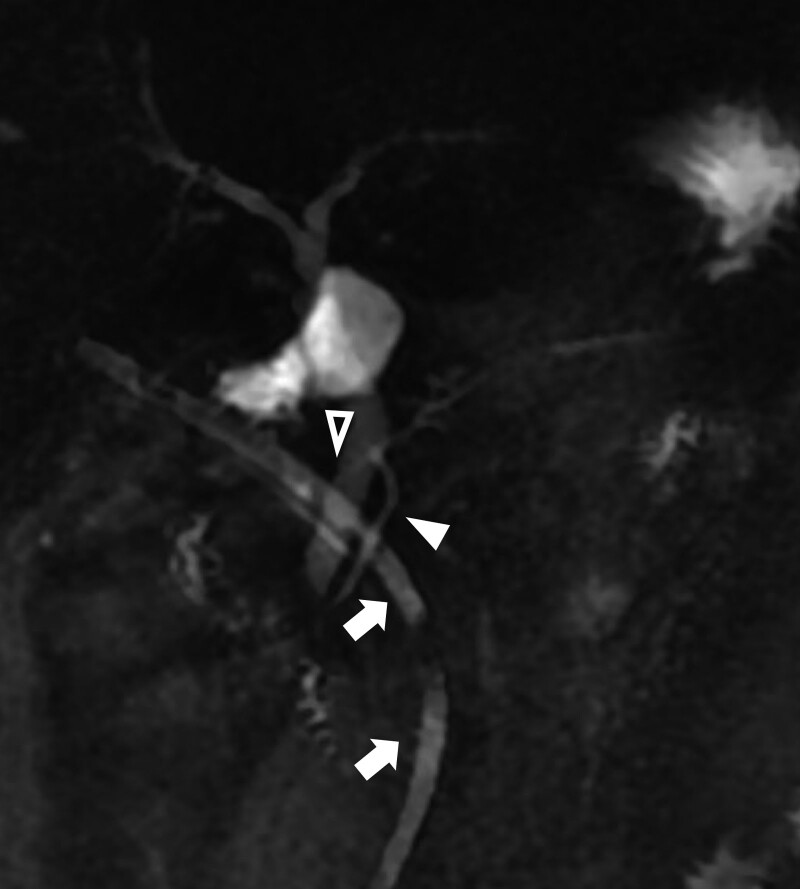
Post-operative MRCP (POD 15). The draining tube is in situ (white arrow), and the ducts of Santorini (hollow arrowhead) and Wirsung (white arrowhead) are both visible. Intersection of the duct of Wirsung and the dorsal duct is highlighted (red arrowhead). Surgical damage to the duct of Santorini, which was almost inevitable due to its path, was considered the cause of post-operative fistula. CT = computed tomography, MRCP = magnetic resonance cholangiopancreatography, POD = postoperative day.
